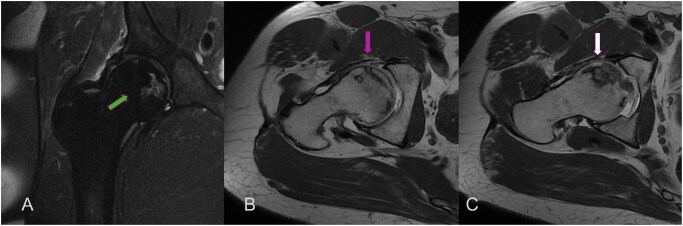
Patient presented with hip pain. MR Arthrogram was performed for the clinical question of labral tear. MRI conformed the anterior labral tear. Incidental AVN of the femoral head was also seen.
